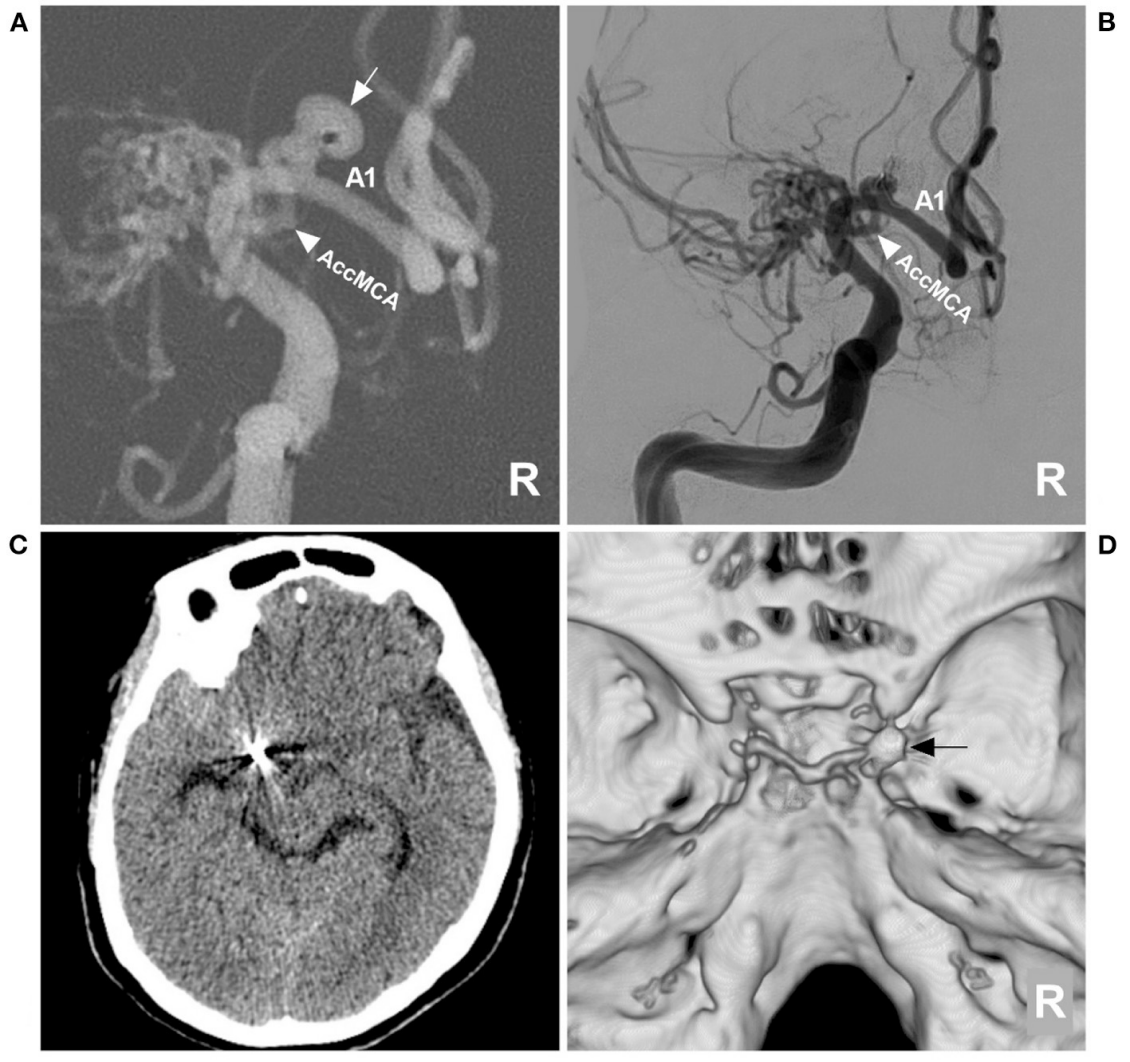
Aneurysm coiling and follow-up CT. (A) DSA roadmap navigation of the right ICA showing that the microcatheter was positioned into the aneurysm sac (arrow); the arrowhead indicates the AccMCA. (B) Postoperative DSA of the right ICA showing that the aneurysm was completely coiled and that the AccMCA (arrowhead) was preserved. (C) Follow-up CT showing normal brain tissue. (D) CT reconstruction showing the coils (arrow). A1, first segment; AccMCA, accessory middle cerebral artery; CT, computed tomography; DSA, digital subtraction angiography; ICA, internal carotid artery; MCA, middle cerebral artery; R, right.
